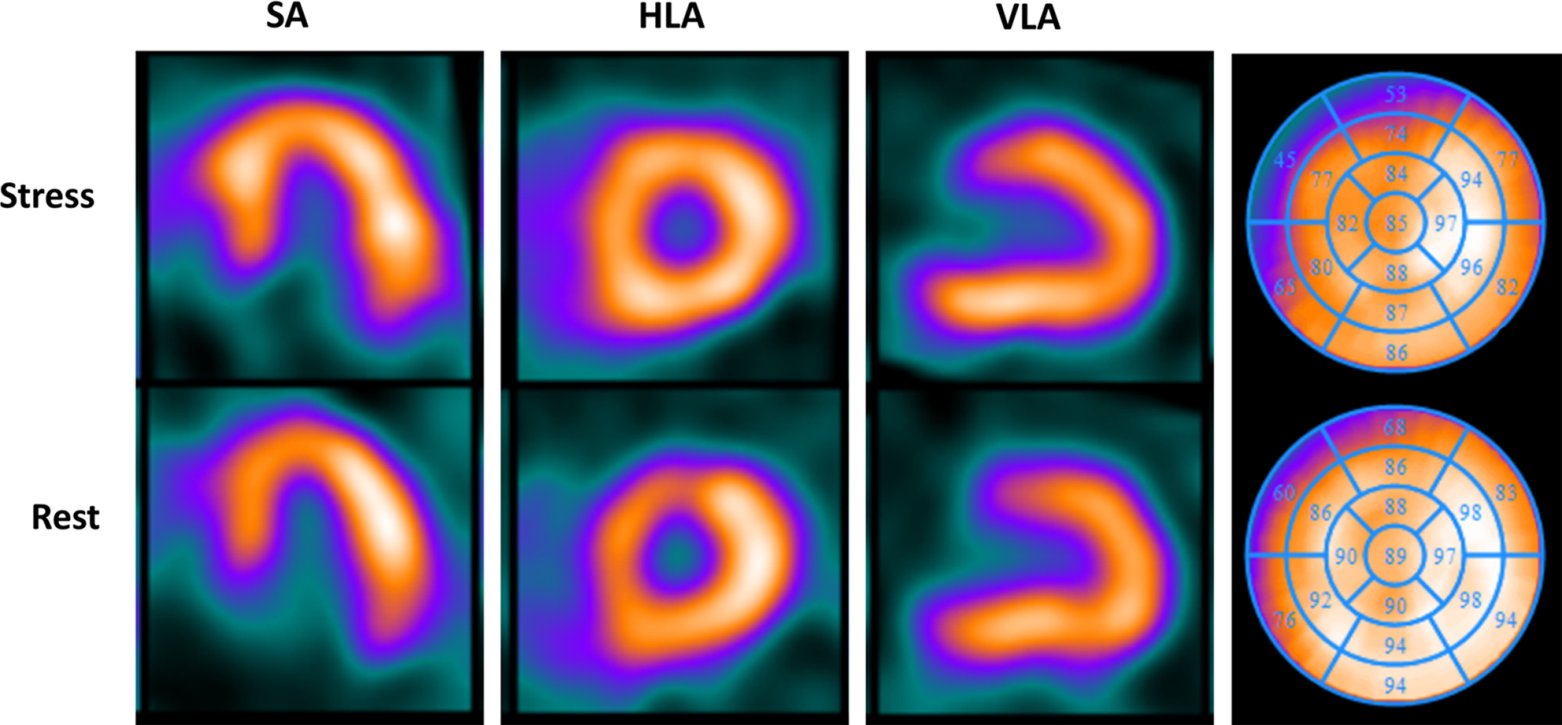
SPECT MPI images in short-axis (SA), horizontal long-axis (HLA), vertical long-axis (VLA), and polar plot demonstrating no perfusion defect at stress and rest
